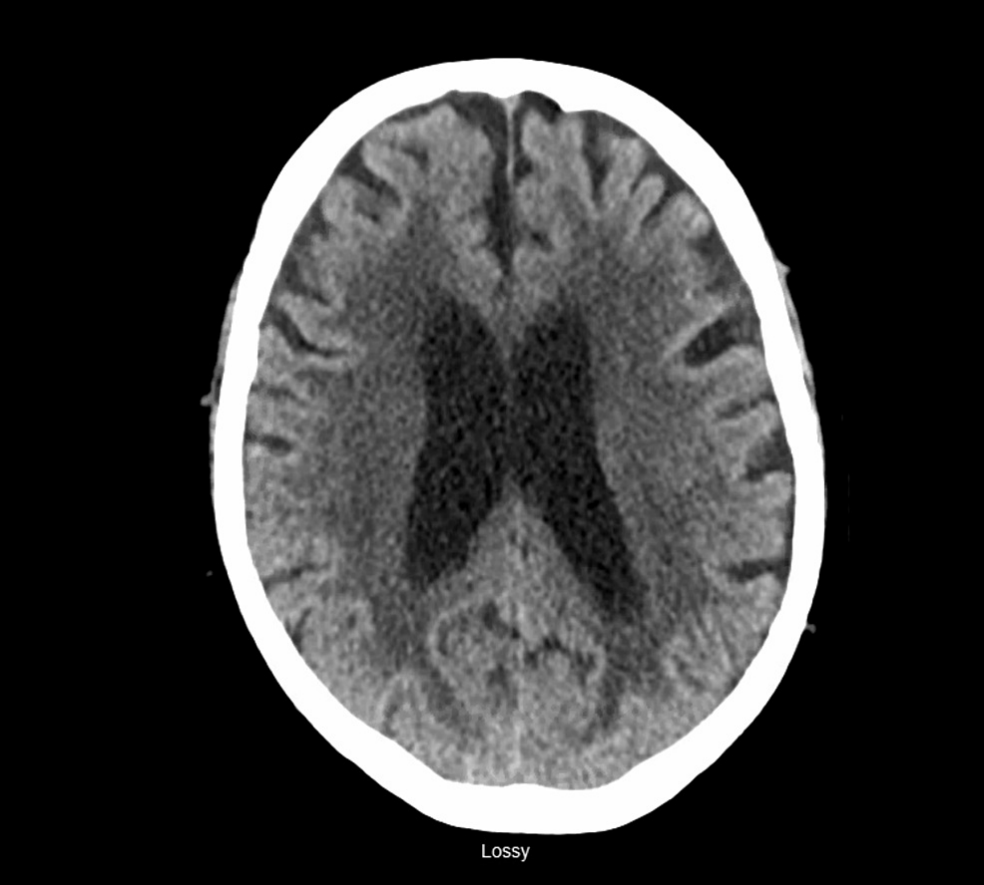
Normal CT scan of the head
